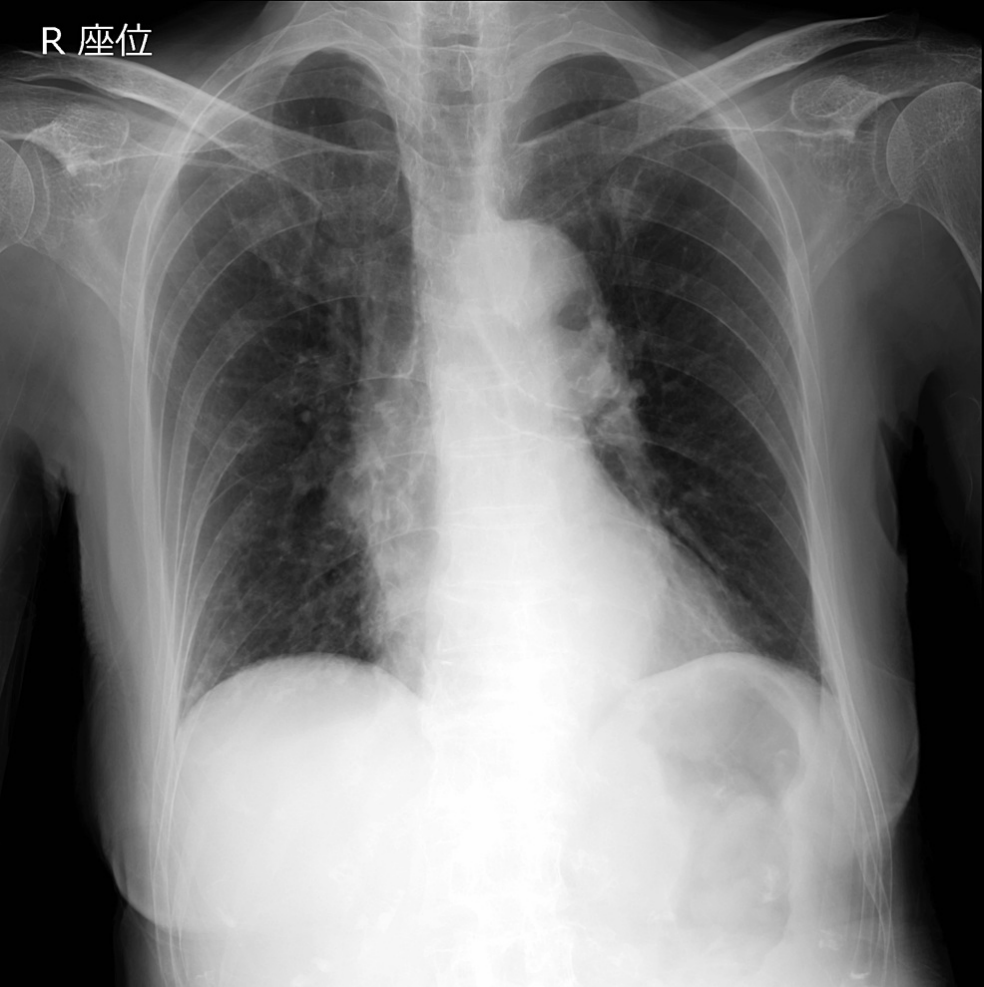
Chest x-ray showed no remarkable findings.
